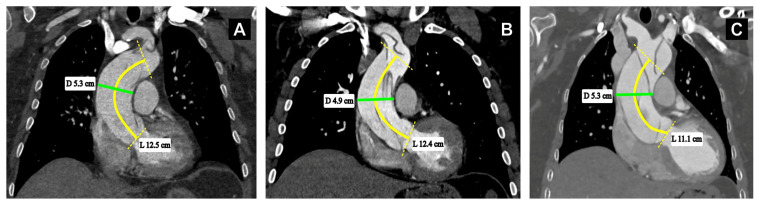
Representative computed tomography angiography (CTA) images highlighting differences in ascending aortic (AsA) length (L) and maximal diameter (D). (A) Representative image of ascending aortic elongation, with an AsA L of 12.5 cm and a maximal AsA D of 5.3 cm. (B) Representative image of pronounced ascending aortic elongation, with an AsA L of 12.4 cm and a maximal AsA D of 4.9 cm. (C) Representative image with an AsA L of 11.1 cm and a maximal AsA D of 5.3 cm. In all panels, the curved yellow line indicates the aortic centerline used for length measurement, the green line labelled D indicates the maximal ascending aortic diameter. The yellow dashed oblique lines indicate the proximal and distal reference landmarks. Ascending aortic length was measured along the centerline from the aortic annulus to the origin of the innominate artery, and diameter was measured in a plane perpendicular to the vessel centerline.
